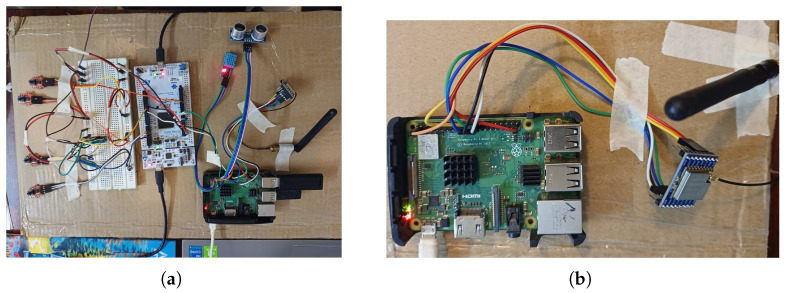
Hardware prototype for secure TPMS telemetry. (a) TX assembles sensor frames, encrypts (AES-128/CBC), authenticates (HMAC-SHA256), and transmits via LoRa P2P. Transmitter node (TX): HX710B → STM32 → Pi (ACU) → LoRa SX1278. (b) RX verifies HMAC, decrypts, and stores only validated measurements, feeding both local dashboards and cloud logging. Receiver node (RX): LoRa SX1278 → Pi (verify and decrypt) → storage/visualization. Auxiliary sensors visible in the prototype (e.g., ultrasonic modules) originate from the continuation of prior experimental research and are retained for contextual or compatibility purposes. However, they are not involved in TPMS sensing, security evaluation, or performance analysis in this study.
